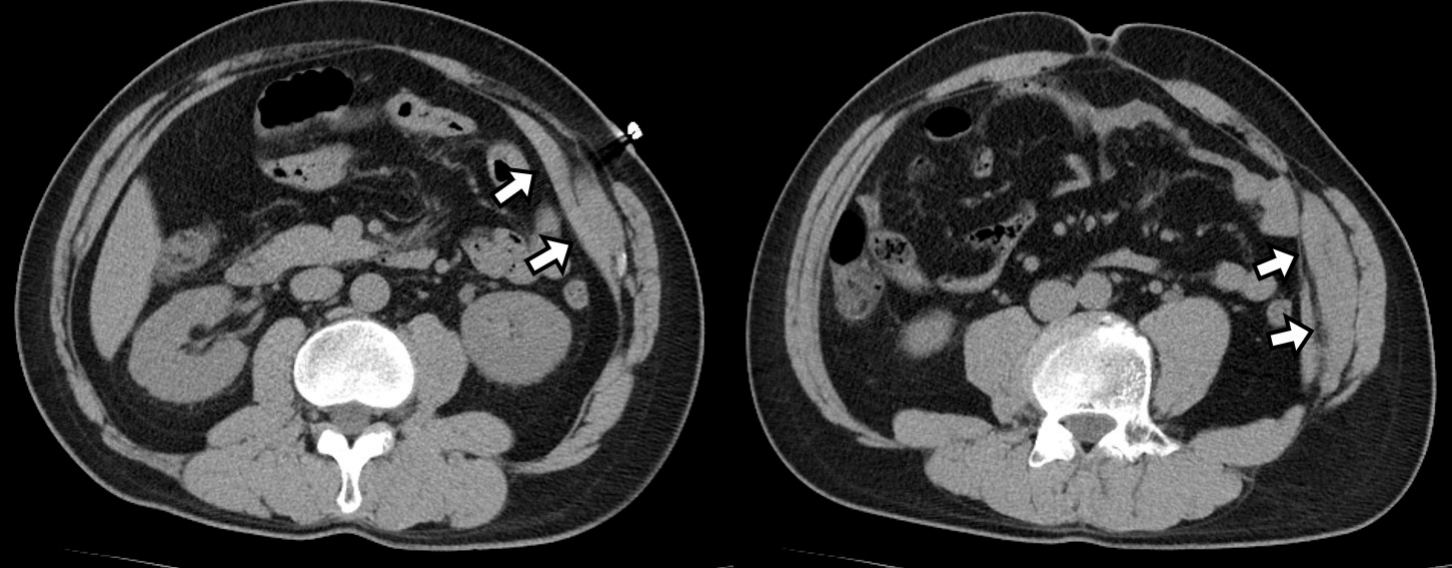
Computed tomography revealed isodensity area along the left internal and external oblique muscles and transversus abdominis muscle, suggesting oblique muscle hematoma.
